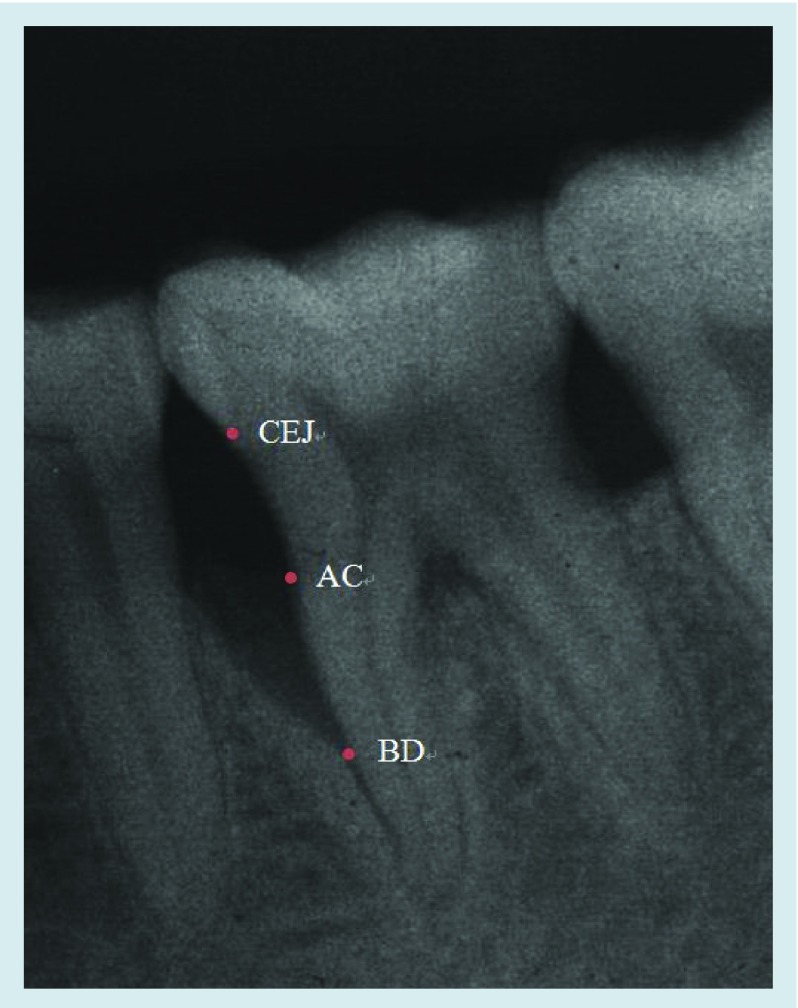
Radiographic landmarks. AC: Alveolar crest; BD: Base of the defect; CEJ: Cemento–enamel junction.
